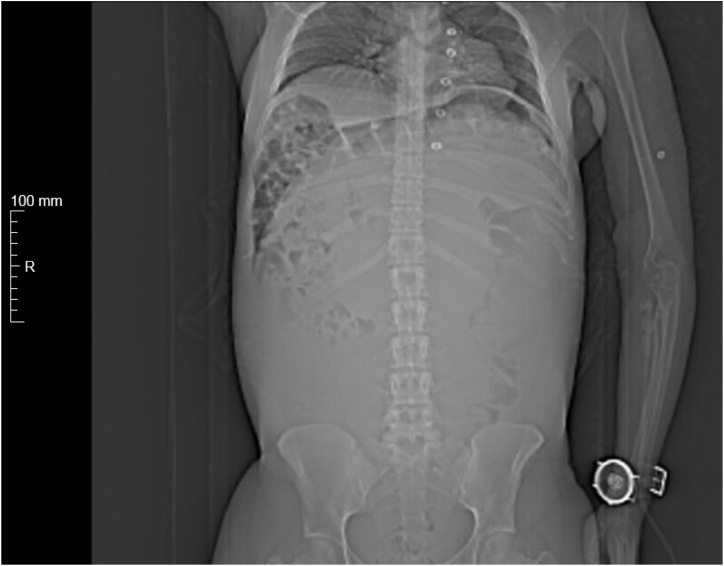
Thoracic, abdominal and pelvic CT showing symmetrical elevation of both diaphragmatic domes and presence of collapsed small bowel in the right upper abdominal quadrant.
